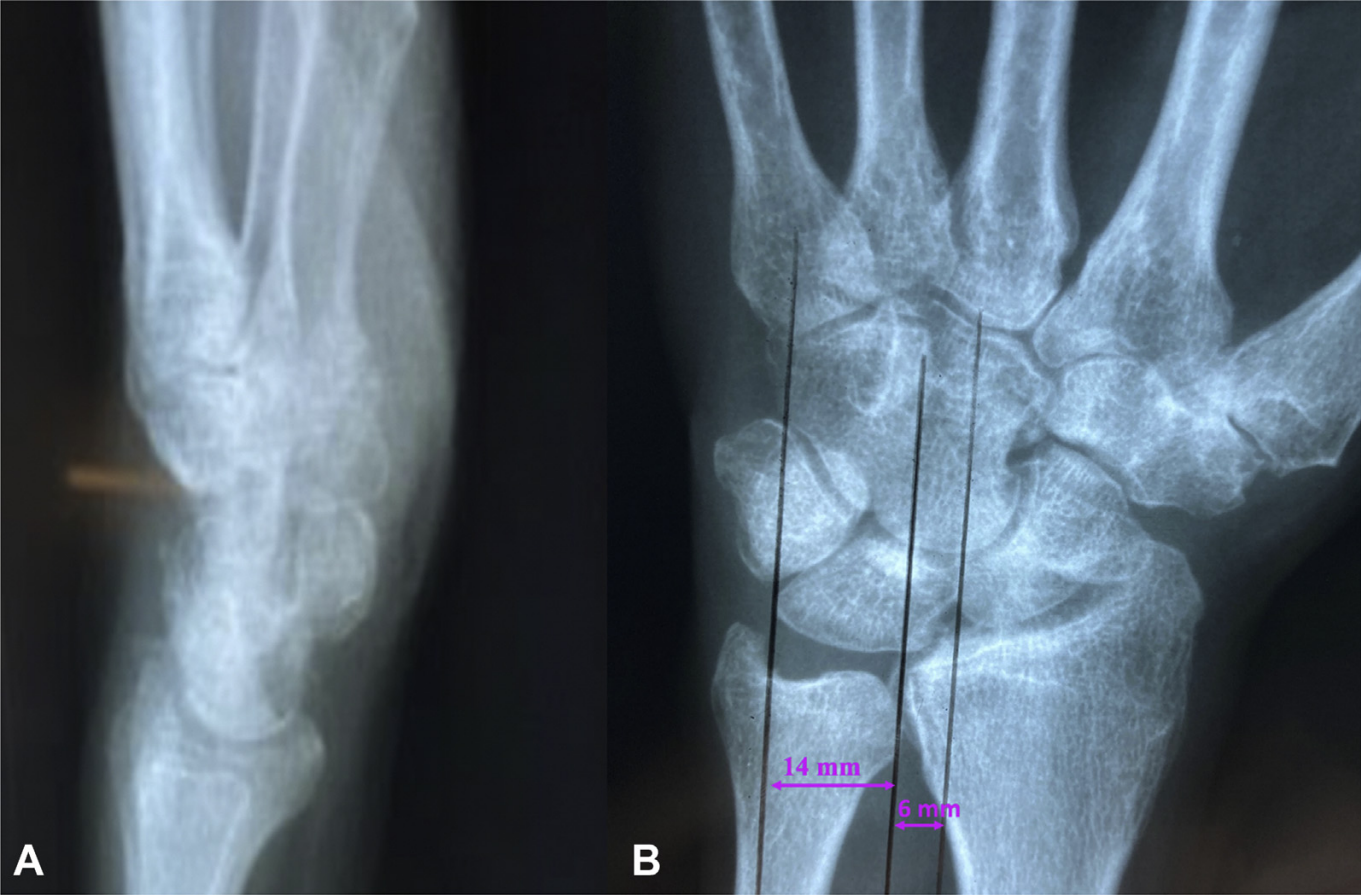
Radiographs of the left wrist taken at 6 months after the initial injury. A Lateral view. B PA view. Lunate uncovering 70%.
